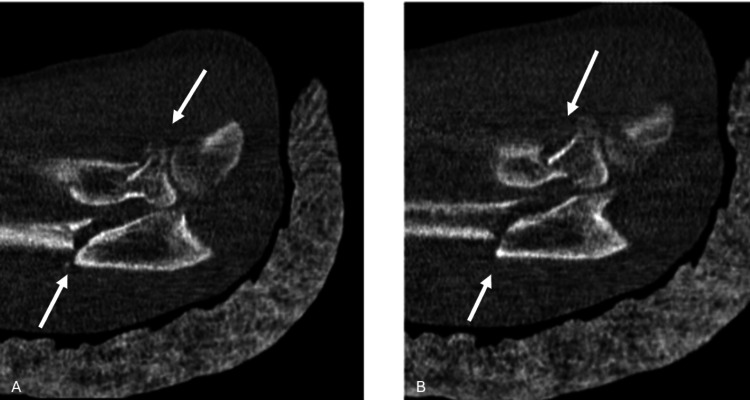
Coronal section of the computed tomography images of the patient. A and B demonstrate the fractures at the proximal ulna and radial head subluxation White arrows indicate the fractures at proximal ulna and radius.
